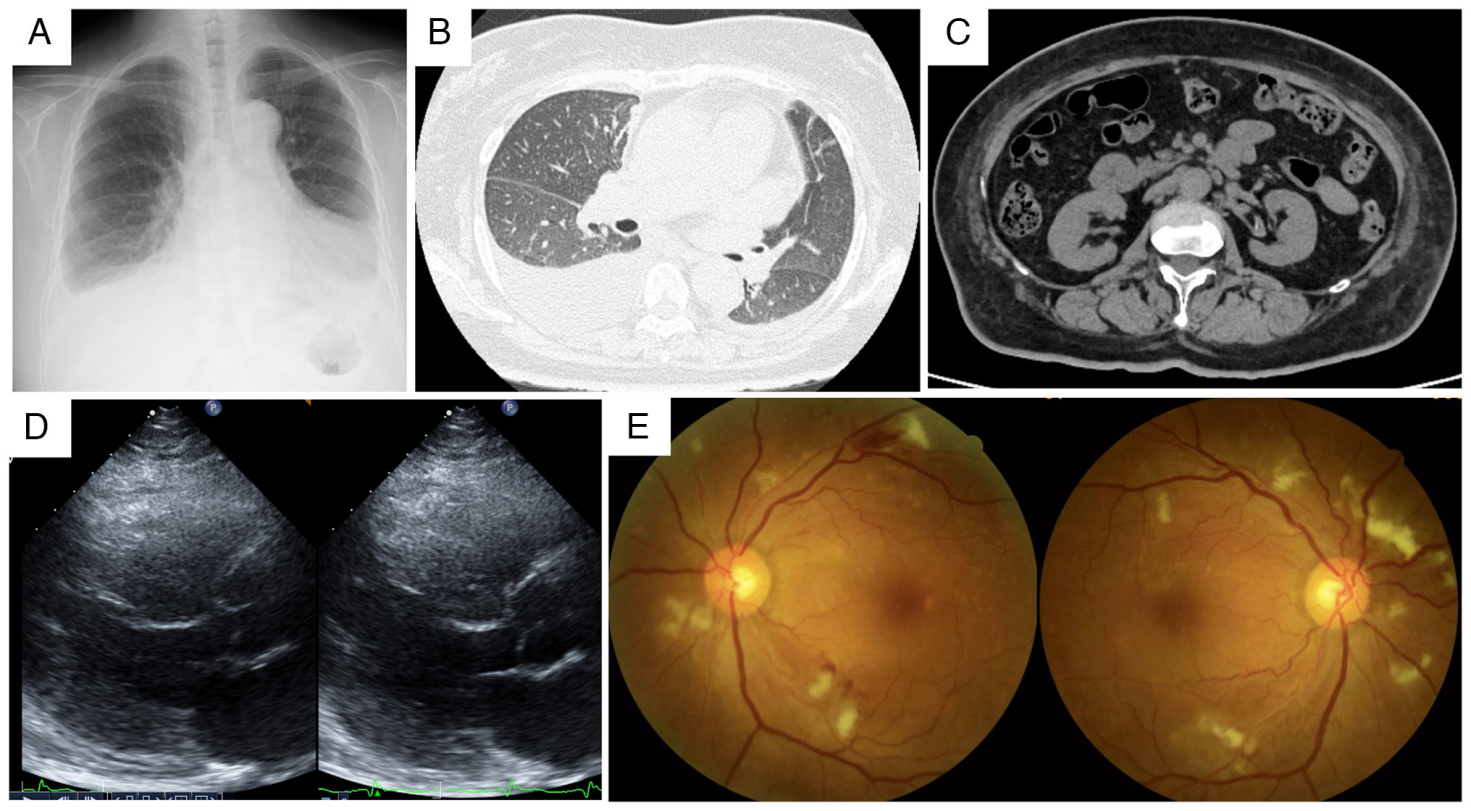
Radiological and imaging findings on admission. (A) Chest radiograph showing cardiomegaly with bilateral pleural effusion, predominantly on the right side. (B) Chest computed tomography image showing bilateral pleural effusion without significant parenchymal abnormalities. (C) Abdominal computed tomography showing subtle bilateral renal atrophic changes. (D) Transthoracic echocardiography revealing diffuse left ventricular hypokinesis with a reduced ejection fraction of 42.5%. (E) Fundoscopic examination confirming hypertensive retinopathy with hemorrhage and exudates.
